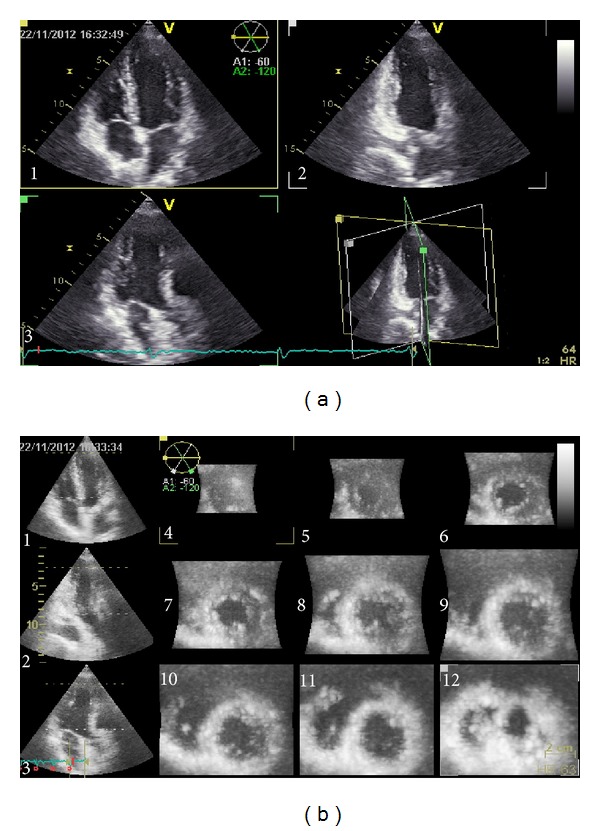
Picture (a) showing a triplane acquisition of the LV (numbers 1, 2, and 3, resp., represent the apical 4 chamber, 2 chamber, and apical long-axis views); picture (b) showing a 12-slice reconstruction of the LV (numbers 1, 2, and 3 as in picture (a); numbers from 4 to 12 showing a sequential reconstruction of short-axis views from the apex to the base of the LV).
